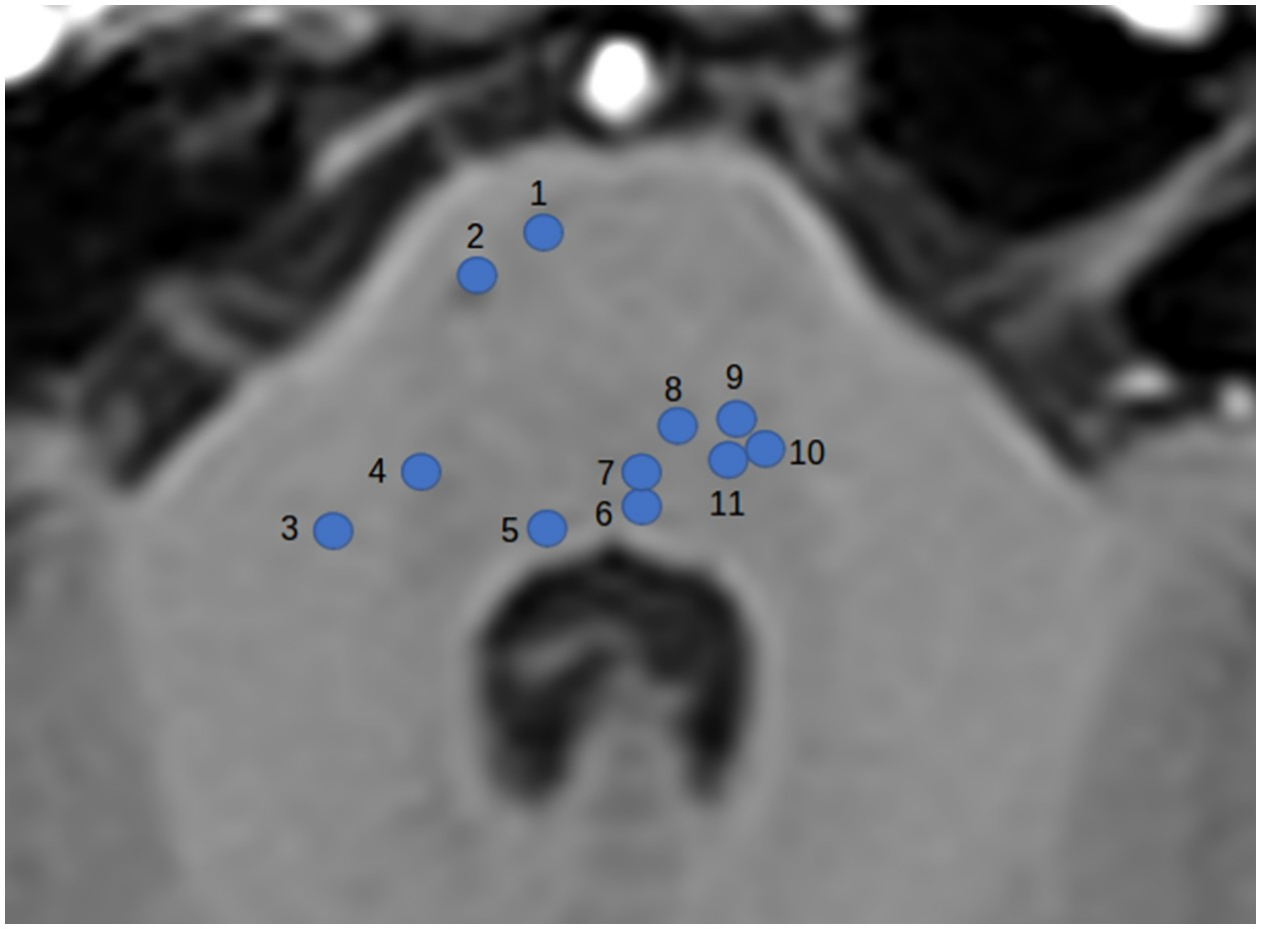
Axial T1 MRI image of the pons with location representation of some of the injured white matter fiber tracts detected by anatomical analysis. 1: Frontopontine tract; 2: Corticospinal tract; 3: Middle cerebellar peduncle; 4: Nucleus of trigeminal nerve; 5: Nucleus of abducens nerve; 6: Medial longitudinal fasciculus; 7: Tectospinal tract; 8: Medial lemniscus; 9: Lateral lemniscus; 10: Rubrospinal tract; 11: Central tegmental tract.
